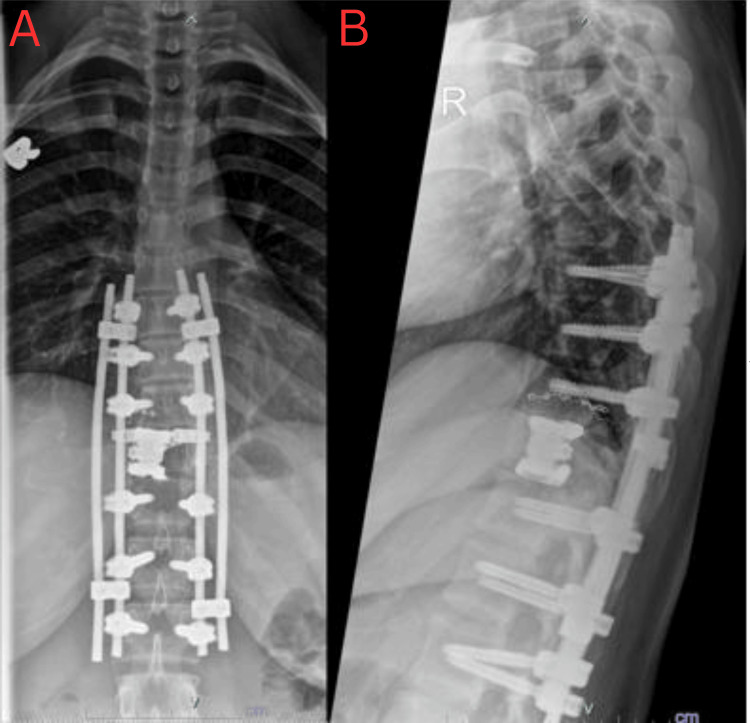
The post-operative thoracic spine X-ray in anteroposterior (A) and lateral (B) views.
